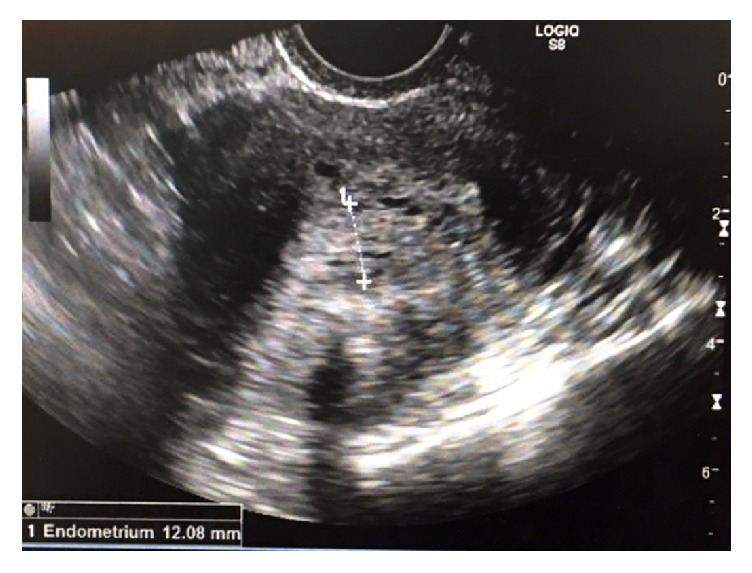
Ultrasound imaging of uterus demonstrating a 12.1 mm endometrial stripe.
